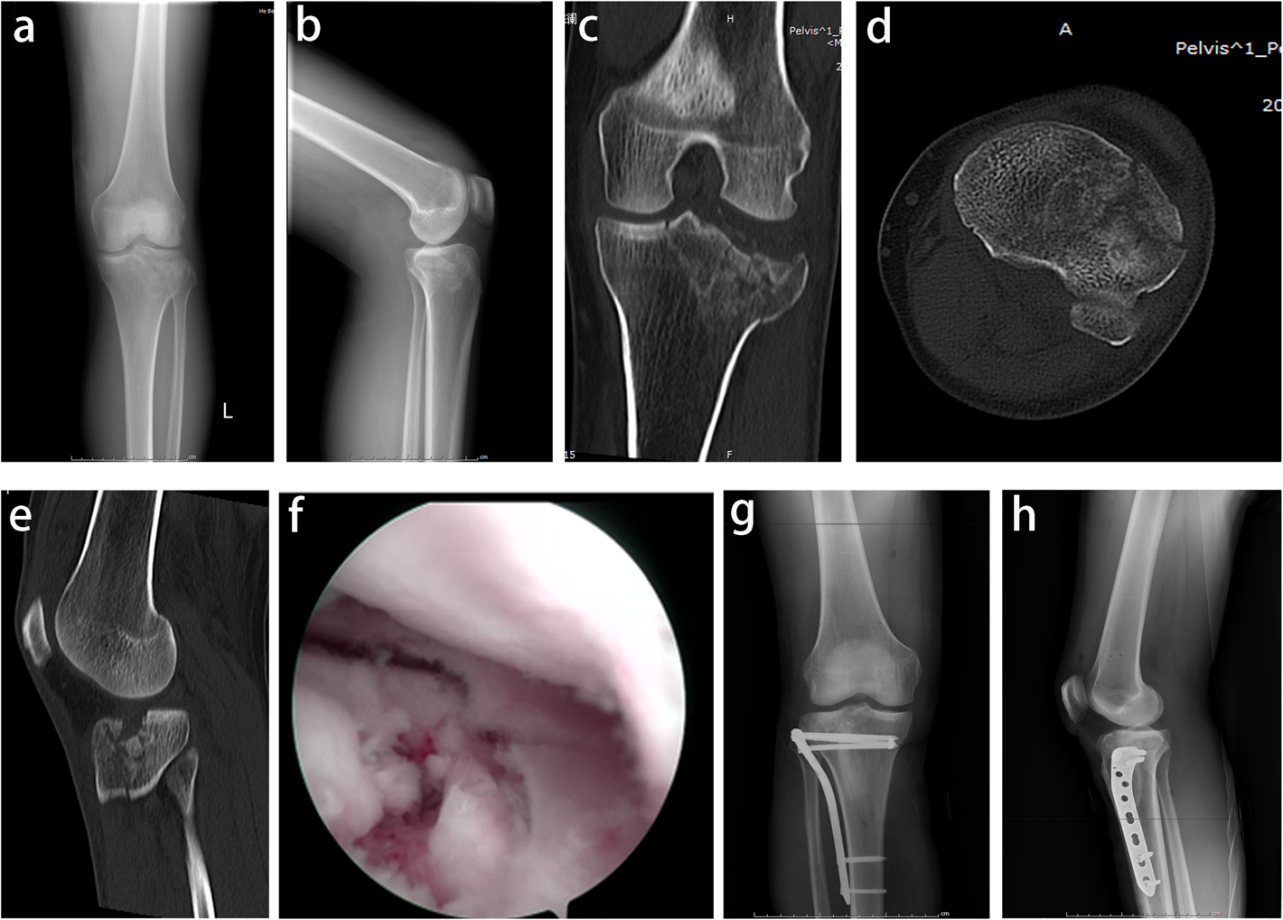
A case of Schatzker type II tibial plateau fractures, male, 47 years old. a, b Preoperative radiographs of the injured knee. c Preoperative coronal CT scan shows the lateral tibial plateau fracture, increased width of the plateau, and articular depression. d Axial CT scan shows tibial plateau fracture involved the anterolateral column. e Sagittal CT scan shows articular depression concentrated in the anterolateral plateau. f Arthroscopic findings intra-operatively shows the radial meniscal tears associated with tibial plateau fracture. g-h Post-operative radiographs
